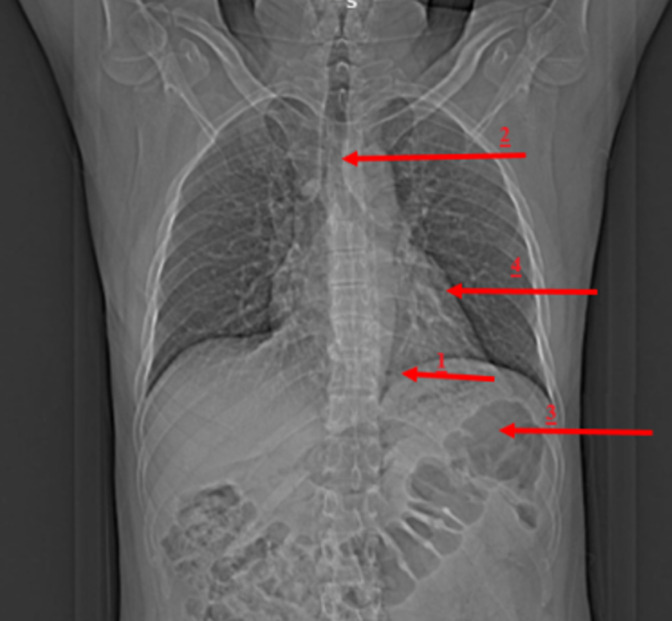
CT image of depicting herniation of abdominal contents into the thoracic cavity and shift of mediastinum towards right side
